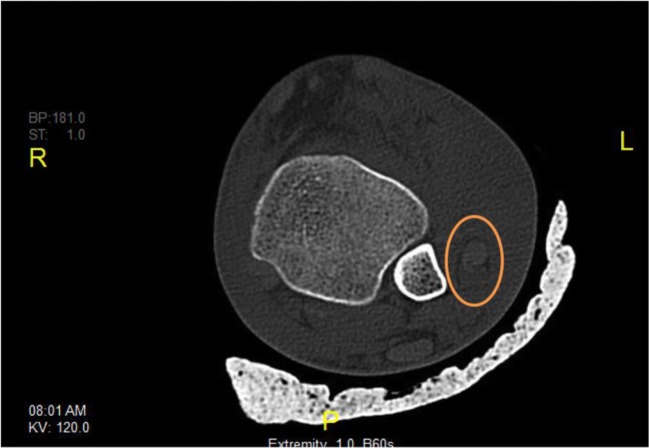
Axial cut from CT scan of a left ankle showing anterior dislocation of peroneal tendons (circled)
